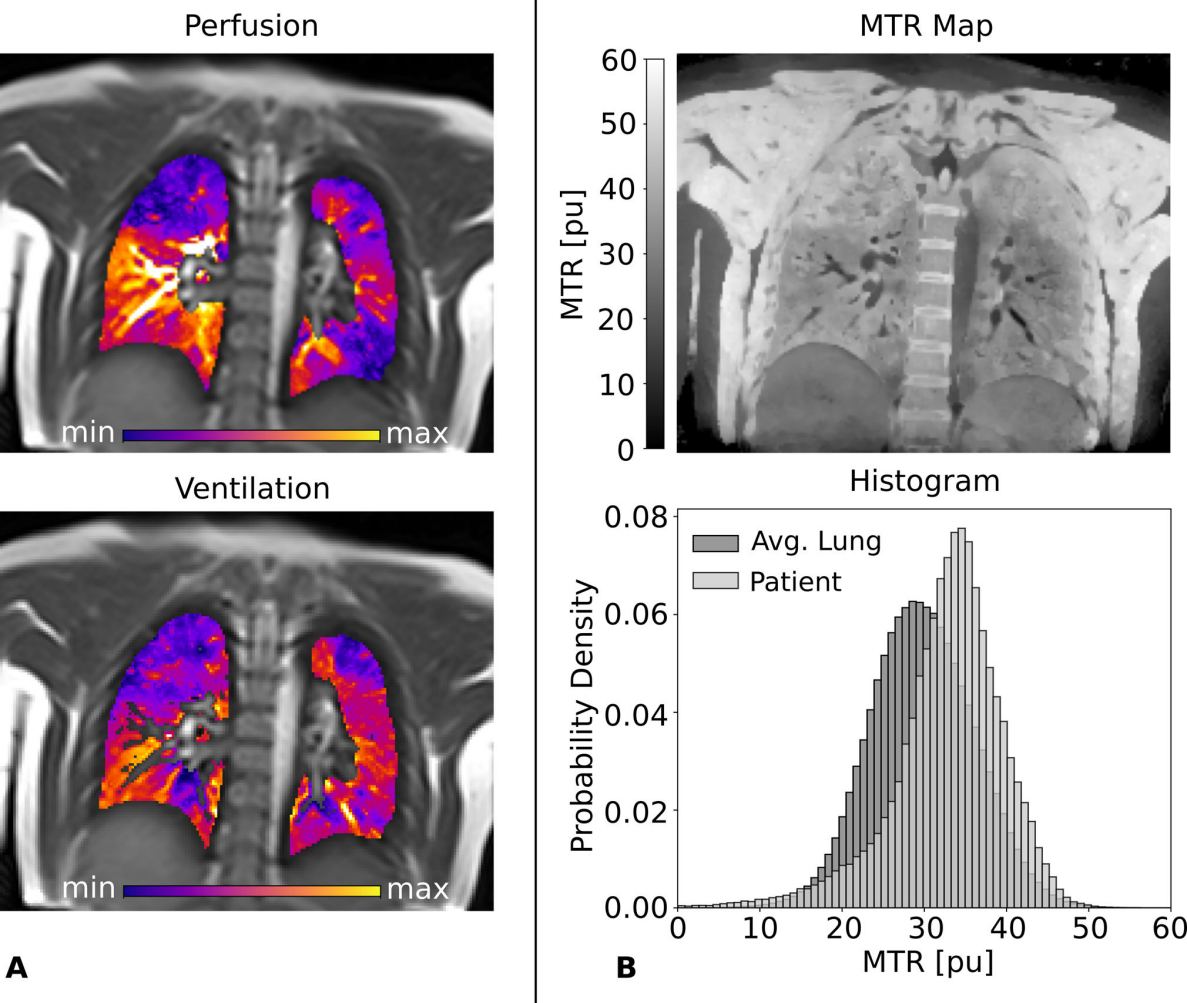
Cystic fibrosis patient: (A) Perfusion‐weighted MP MRI map and ventilation‐weighted MP MRI map and (B) MTR map and whole lung MTR histogram for the patient in light gray and collective data of healthy volunteers, representing an average healthy lung, in dark gray.
